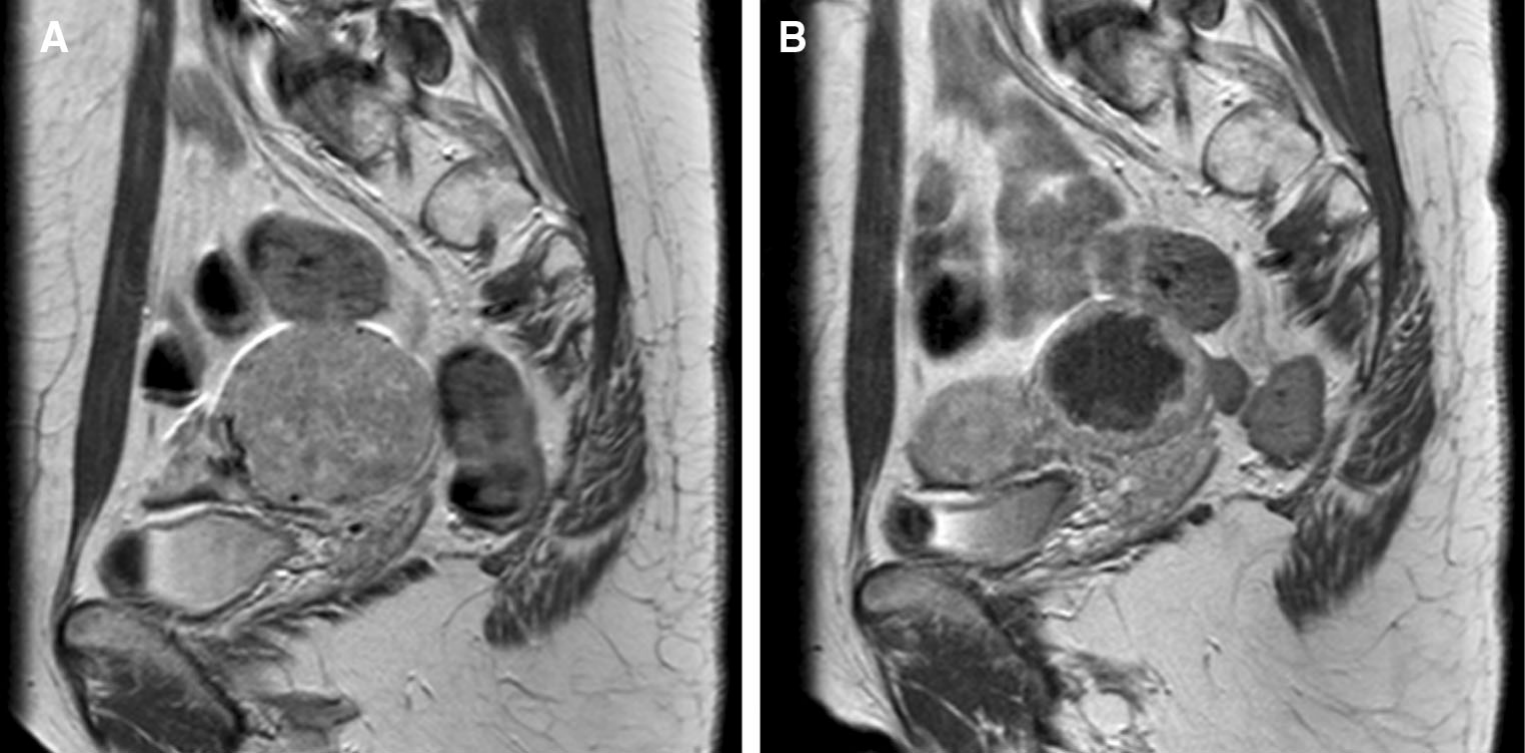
MRI (*SAG T1* − *TSE* − *HR contrast* +) imaging of a solitary cervical leiomyomata. *Note* Patient no. 2, MR images (sagittal T1 − TSE − HR + contrast) of a single cervical leiomyomata in a 37-year-old patient with heavy menstrual blood loss and a wish to conceive. Cervix with a broad-based leiomyomata on the left. A The cervical leiomyomata (106 cm^3^) with full enhancement prior to UAE. B MR imaging 3 months after UAE showed 95% infarction with an irregular enhancing rim. Unilateral left-side UAE with 2cc500, 2cc700 and 3cc900 µm microspheres Embozene™; 47% volume reduction (56.4 cm^3^)
